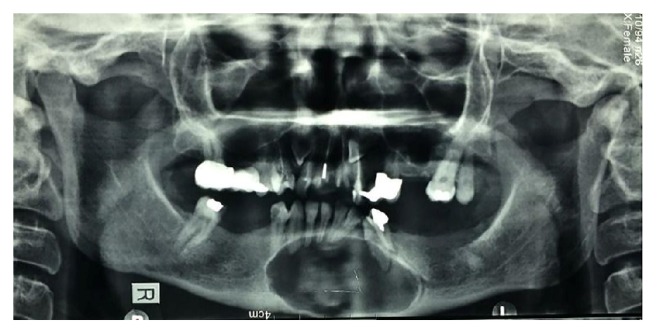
Panoramic radiograph shows a single large radiolucent lesion with well-defined border.
